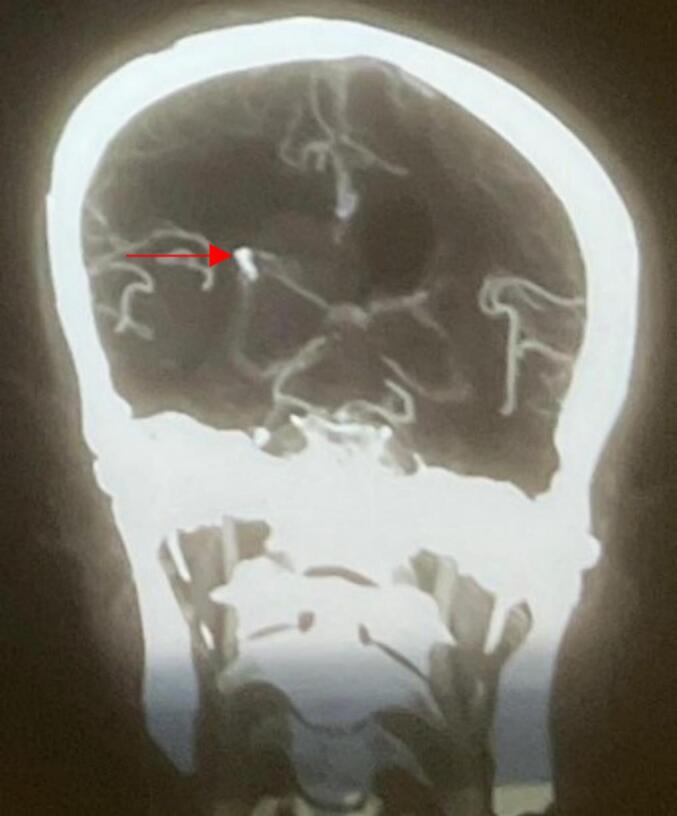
CT cerebral angiography shows distal right middle cerebral artery suspected aneurysm (red arrow).
